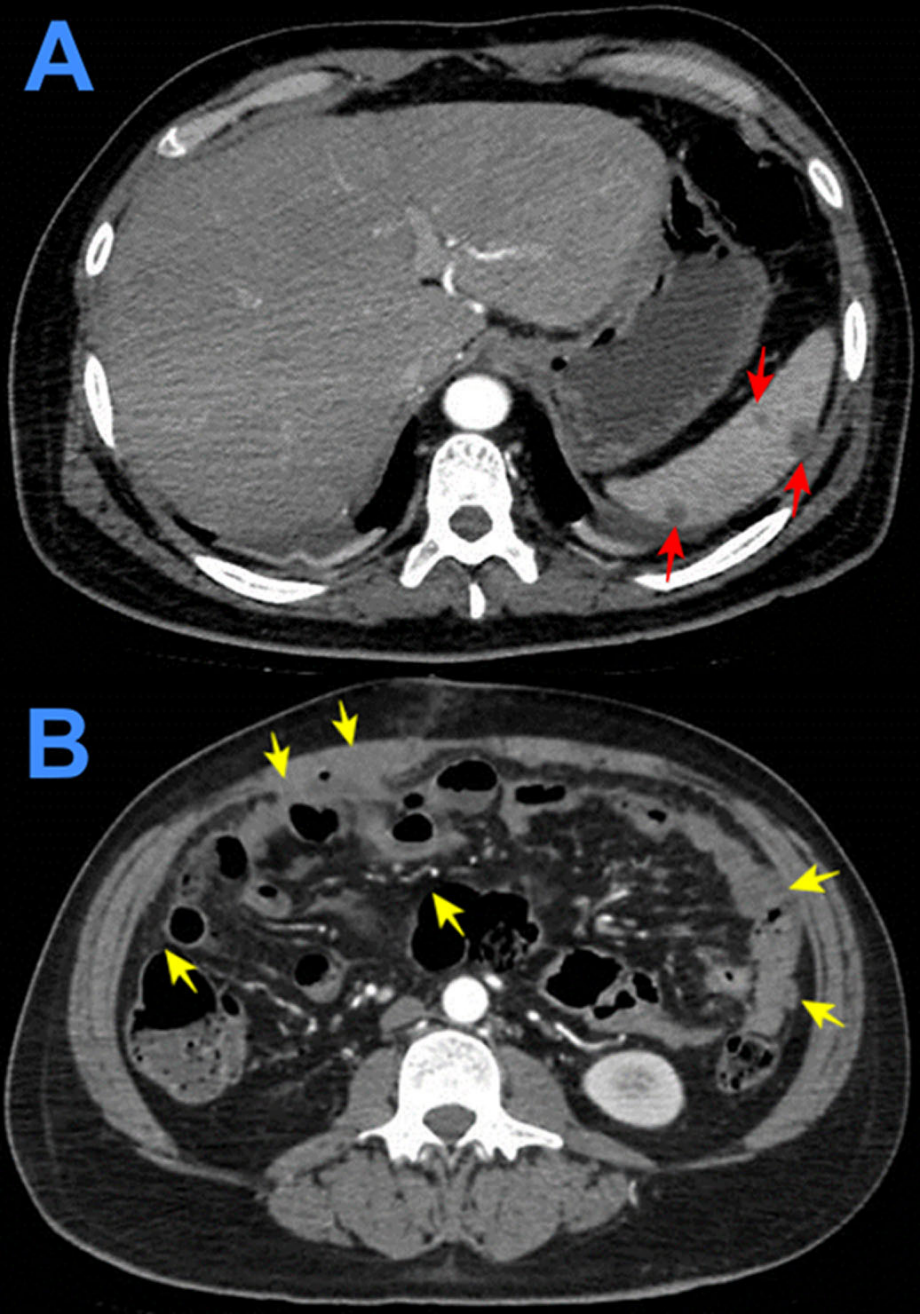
Contrast enhanced computed tomography showing solid focal hypodense areas of <20 mm in diameter that do not enhance with contrast (A). Diffuse thickening of parietal peritoneum with multiple hypodense nodular implants with well-defined borders; inflammatory changes in both iliac fosses; and scarce free fluid in the paracolic gutters and paravesical spaces (B).
